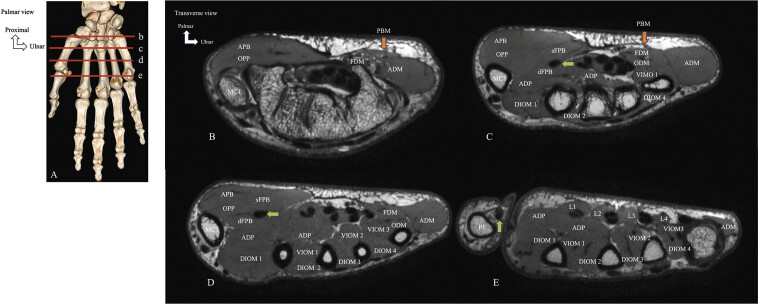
Normal appearance of the intrinsic muscles of the hand on transverse MRI. Levels of the different cross sections, CBCT of the hand, volumetric rendering reconstructions (
